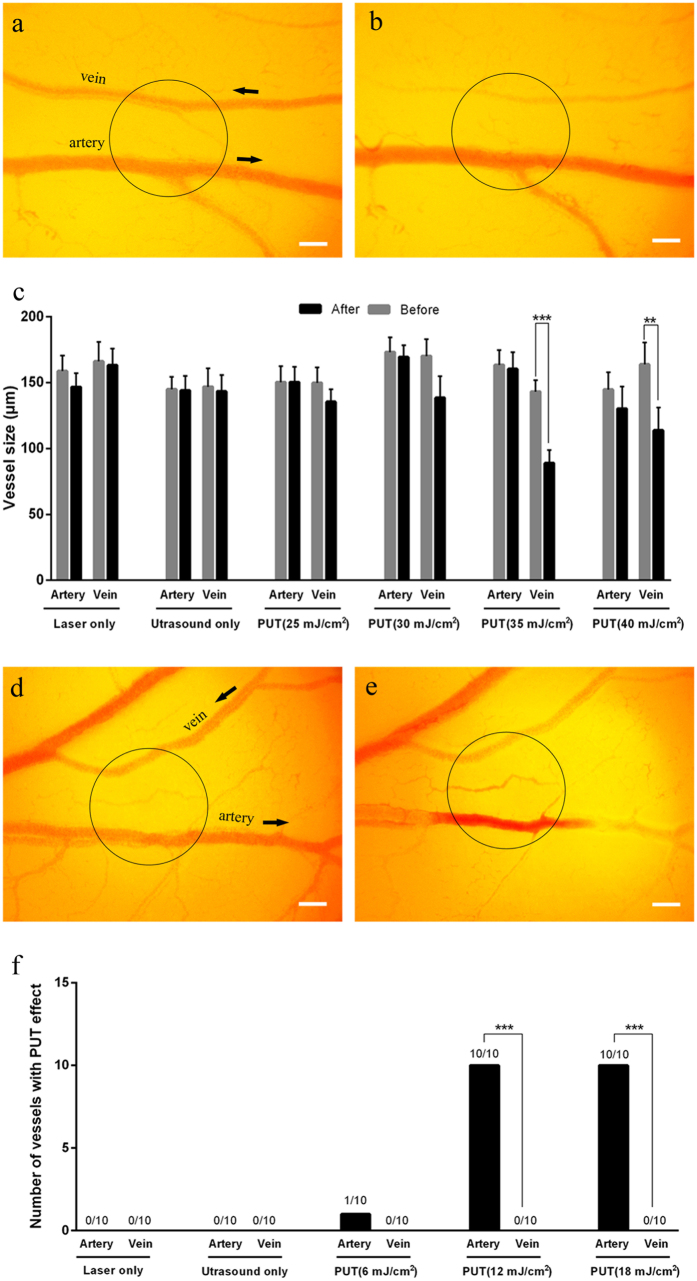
Selective treatment of veins or arteries on chicken *yolk sac* membranes using PUT. (a,b) The photographs of a pair of artery and vein before and after a treatment, respectively. PUT was performed with 0.25 MPa ultrasound negative peak pressure and 35 mJ/cm^2^ laser fluence at 578 nm. (c) Changes in the vessel diameters after different treatments. The changes of the vessel size were only statistically significant in the veins of the PUT groups with 35 mJ/cm^2^ and 40 mJ/cm^2^ laser fluence (n = 10 for each group). Vertical bars indicate standard deviation. The laser wavelengths in (c) were all at 578 nm. (d,e) The photographs of a vein/artery pair before and after a treatment, respectively. The PUT treatment was performed with 0.45 MPa ultrasound negative peak pressure and 12 mJ/cm^2^ laser fluence at 650 nm. (f) The number of veins and arteries that responded to each treatment (n = 10 for each group). Response to treatment was defined as either rupture or occlusion of a blood vessel. The laser wavelengths in (f) were all at 650 nm. The treated area is circled and black arrows indicate the orientation of blood flow. *p* < 0.01; p* < 0.001. Scale bar: 200 μm.
